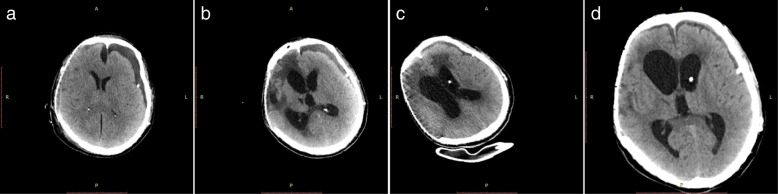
Brain CT scan after cranioplasty and ventriculoperitoneal shunt. (a) Brain CT scan on the first day after cranioplasty. (b) Follow-up brain CT scan after cranioplasty revealed incomplete resolution of the subdural effusion and re-dilation of the ventricles. (c) Brain CT scan on the first day after ventriculoperitoneal shunt. (d) Follow-up brain CT scan three months after ventriculoperitoneal shunt
